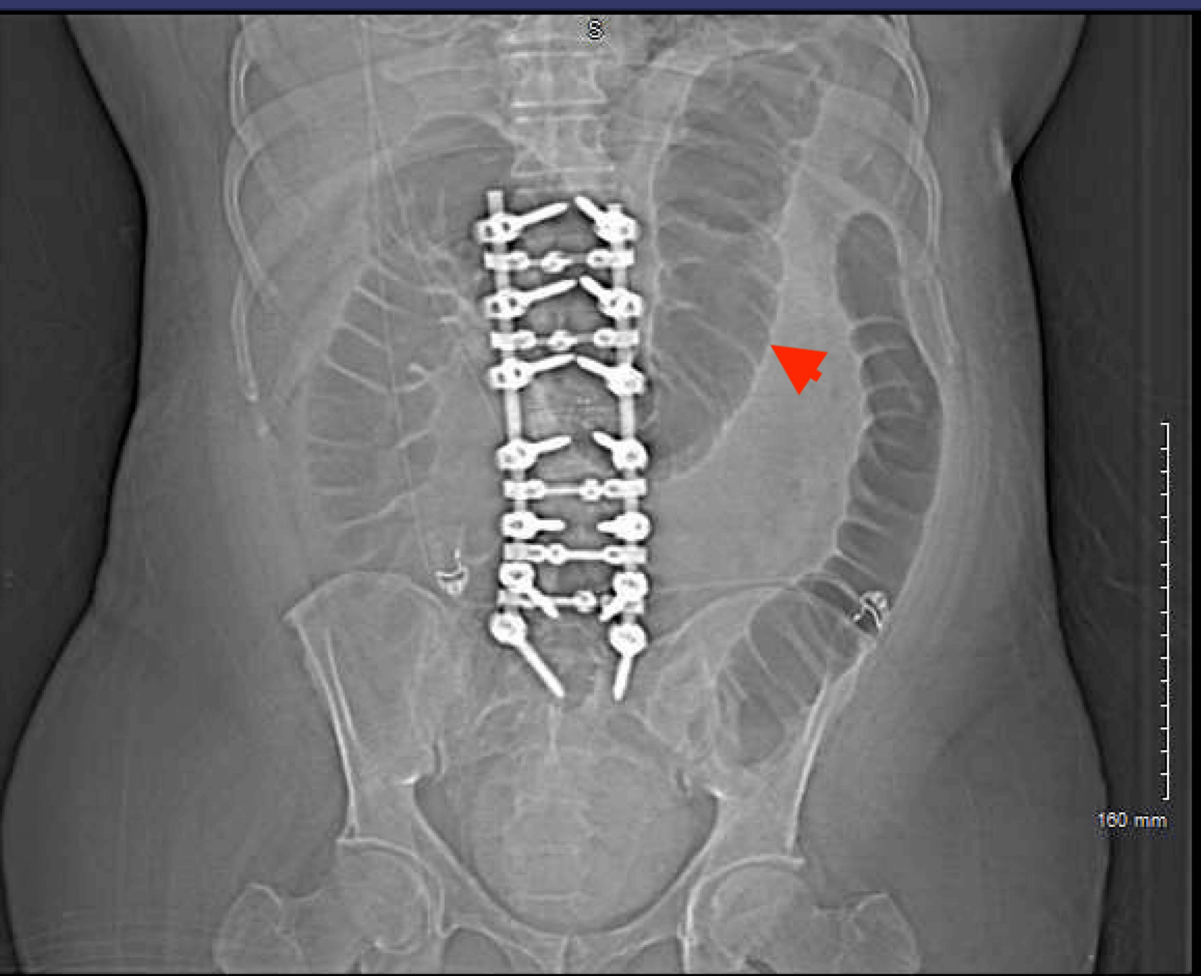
Abdominal CT scan A CT scan of the abdomen confirming the colonic dilation and ileus (red arrowheads)
